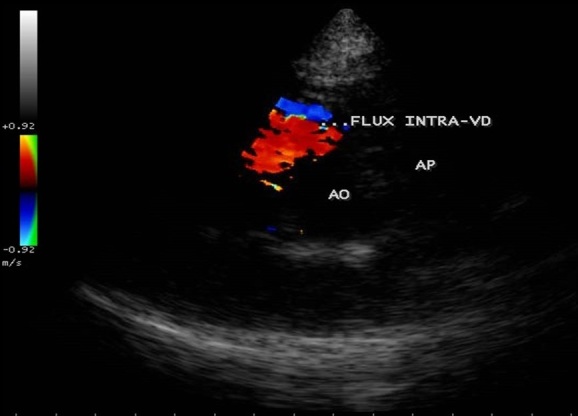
Post-opératoire (3 mois): incidence parasternale petit axe, Döppler couleur avec flux laminaire témoignant d'une absence d'obstruction intra-VD
